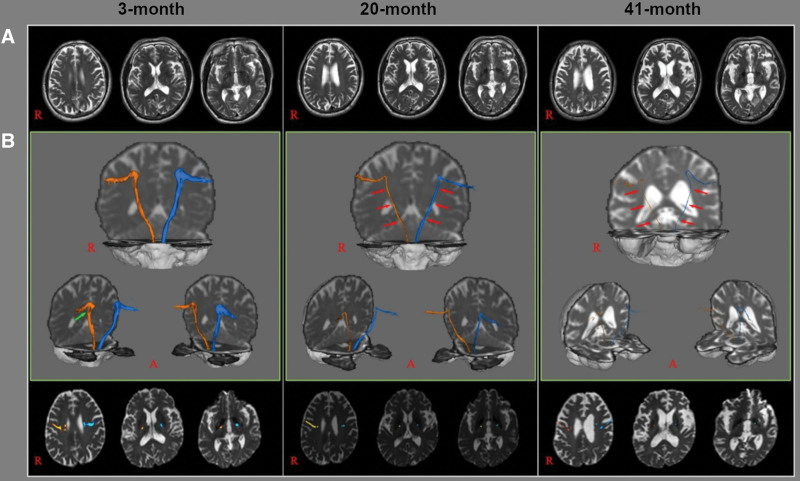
MR images of brain and diffusion tensor tractography (DTT) images for the corticobulbar tract (CBT). (A) Axial T2-weighted image of brain (B) On 3-month DTT, well reconstructed CBT is shown in the left hemisphere; in contrast, the right CBT showed partial tearing (green arrow). Both CBTs in 20-month DTT became thinner (red arrows) compared to 3-month DTT and on 41-month DTT, both CBTs were much thinner (red arrows) than 20-month DTT. CBT = corticobulbar tract, DTT = diffusion tensor tractography.
